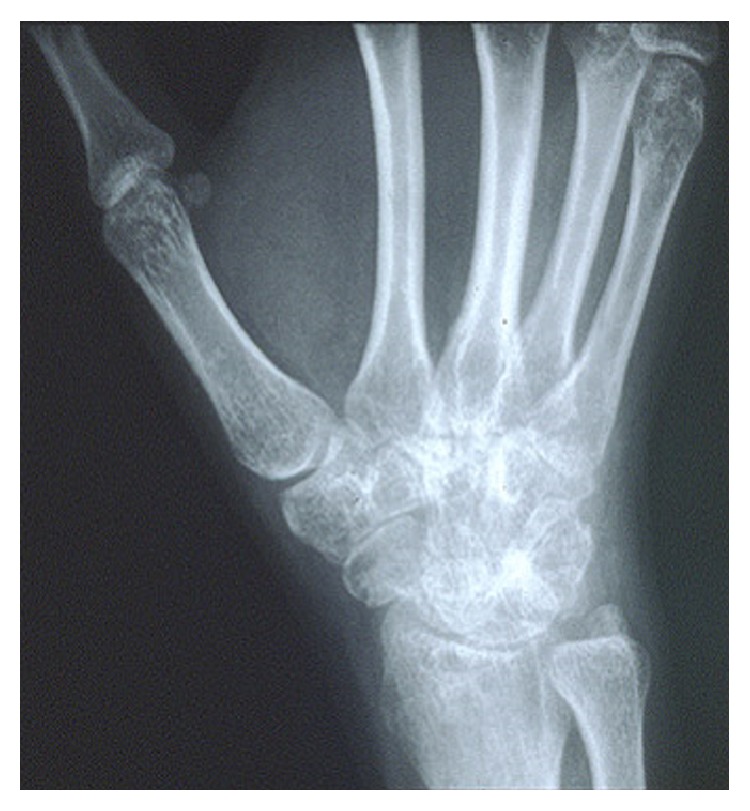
Xray of the right wrist showing diffuse narrowing of the radiocarpal and carpometacarpal joints of the wrist which is a characteristic finding in Still's disease. (Source: http://www.hopkinsarthritis.org/)
